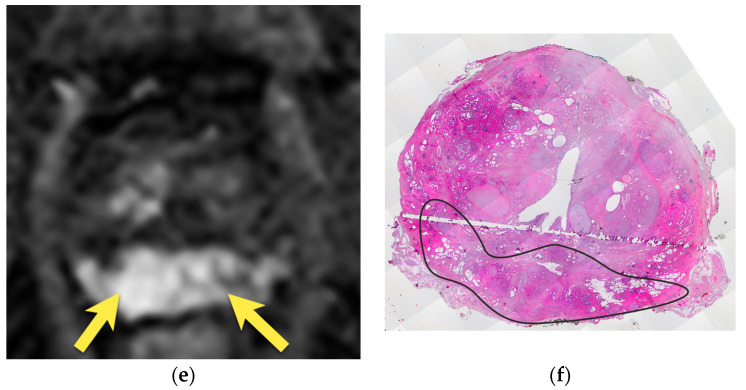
Prostate cancer in the median posterior gland in a patient with PSA 21.36 ng/mL (a) axial and (b) coronal T2-weighted images showing a focal, ill-defined area of low signal intensity located in the median posterior area at the apex and middle third of the prostate (arrows), with marked diffusion restriction on (c) DWI b 2000 image (arrows) and (d) ADC map (arrows); and (e) early enhancement (arrows). The lesion was assessed as PI-RADS 5. (f) After radical prostatectomy, PCa GS 5 + 4 was diagnosed in this location (area outlined with black continuous line).
